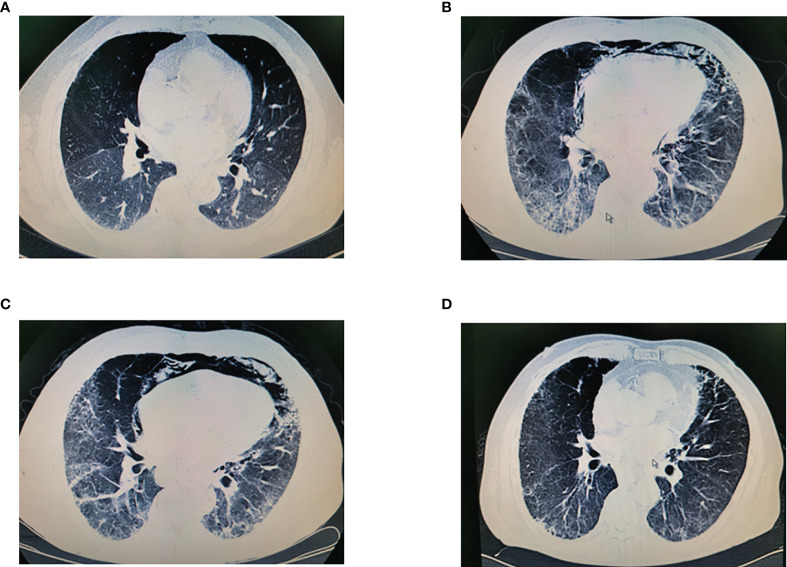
(A) Chest CT scan before surgery. (B) Chest scan on 5th day after operation. (C) Chest scan after application of methylprednisolone for 7 days. (D) Chest scan in 3 months after discharge.
